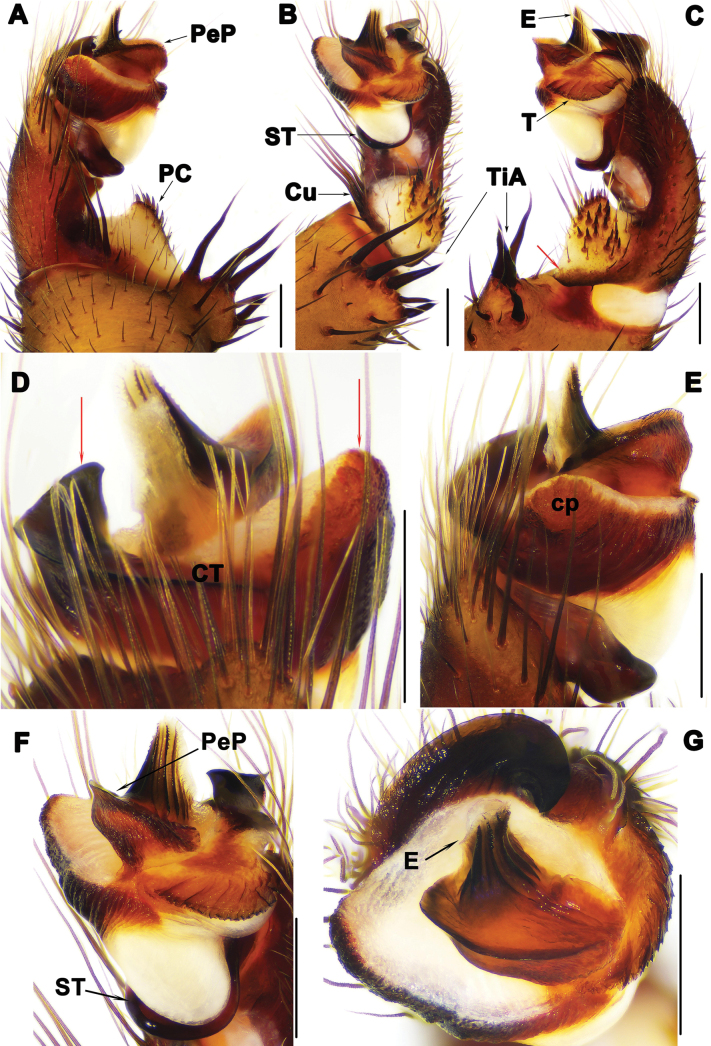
Male palp of *Liphistiuskanpetlet* sp. nov. A, E prolateral view B ventral view; C, F retrolateral view, red arrow shows distal process of PCD dorsal view, red arrows show arched projection and triangular process of CTG distal view. Abbreviations: CT = contrategulum; cp = contrategular process; Cu = cumulus; E = embolus; PC = paracymbium; PeP = paraembolic plate; ST = subtegulum; T = tegulum; TiA = tibial apophysis. Scale bars: 0.5 mm.
